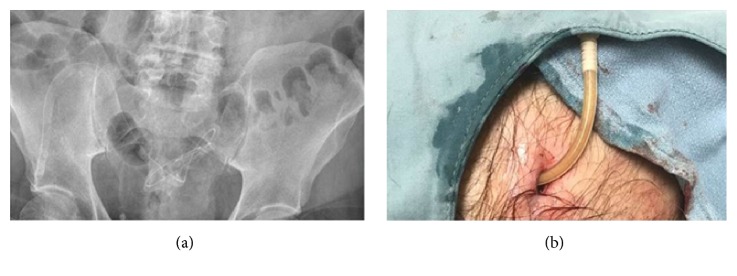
(a) Plain radiograph of the abdomen showing position of catheter prior to exteriorization. (b) Catheter immediately after exteriorization.
